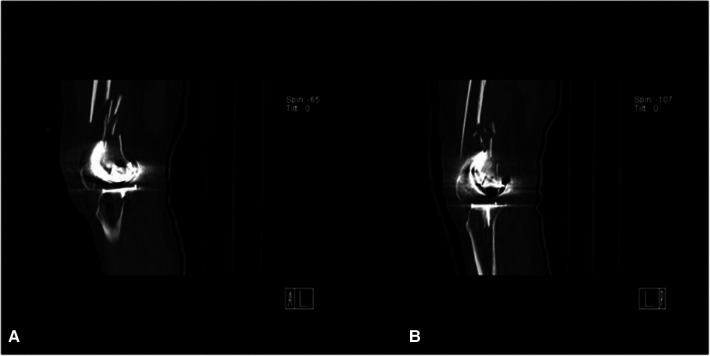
Preoperative sagittal CT of bilateral knees: the right knee (A), the left knee (B).
